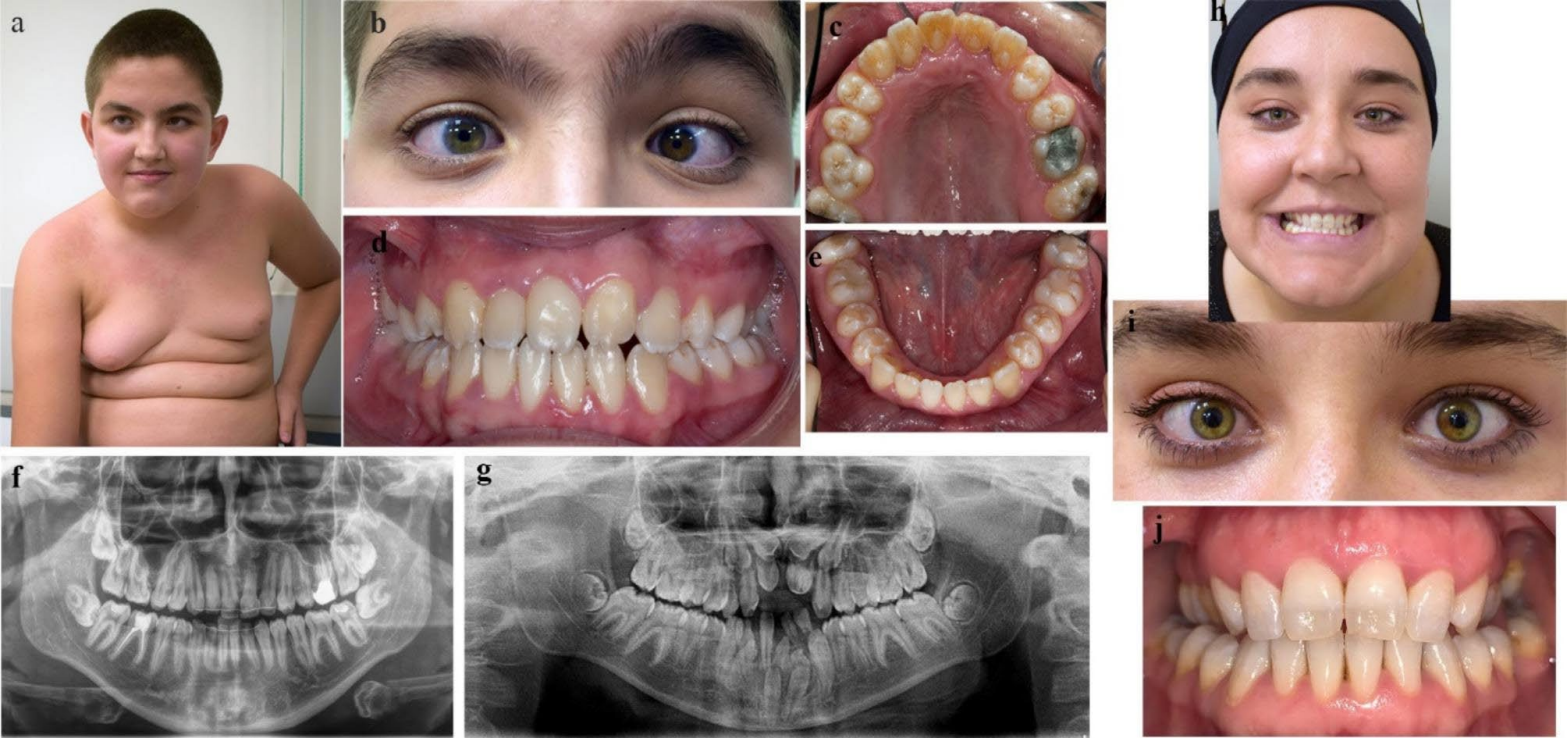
Clinical and radiographic images of affected proband (a-g) and carrier mother (h-j) from Family 1. (a) Note the bilateral gynecomastia and mild dysmorphism with short philtrum, short palpebral fissures, and anteverted pinnae (b) Close-up view of eyes showing bilateral rotatory nystagmus, strabismus on the left eye, and heterochromia. (c-e) Intraoral images at 15 years old demonstrating Hutchinson incisors, bud-shaped molars, talon cusps in maxillary and mandibular incisors, and localized hypomineralised areas of enamel in the incisal edges and cusp tips. (f) Panoramic radiograph at 15 years old (g) Panoramic radiograph at 10 years old showing the supernumerary teeth in anterior maxilla and mandible. (h-i) Facial and eye close-up view of mother. Note the partial heterochromia of both eyes. (j) Intraoral image shows tapered maxillary central incisors and increased translucency of enamel in nearly half of the incisors’ crowns
